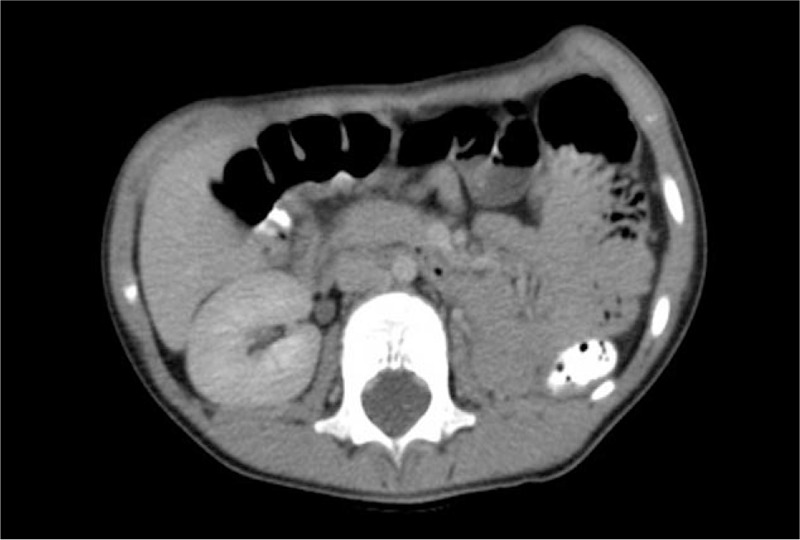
CT scan obtained at the patient's age of 4 years and 10 months. The right kidney appeared normal after partial resection of the lower pole. There was no dilatation of the right renal pelvis after high uretero-ureterostomy.
